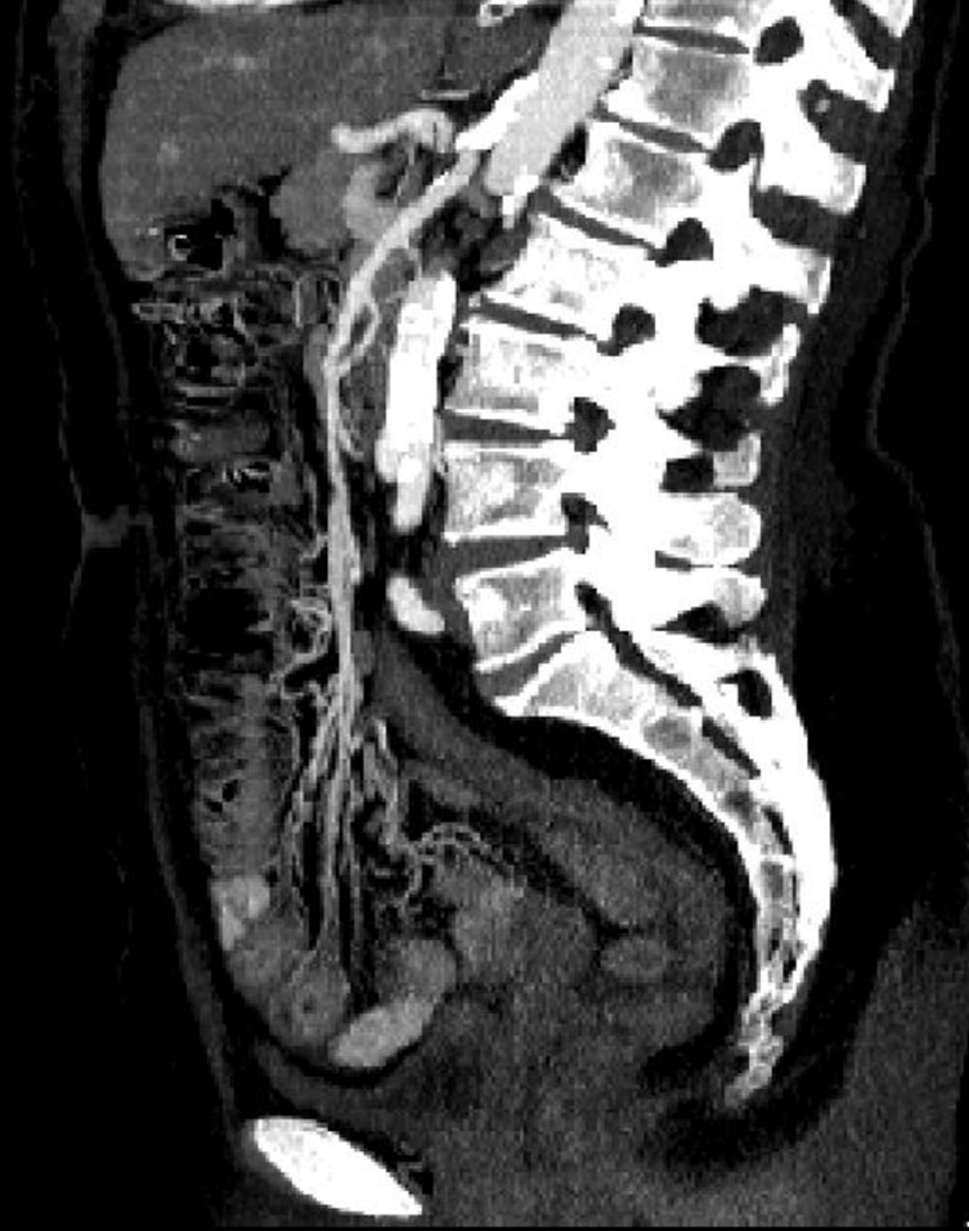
Acute arterial mesenteric ischemia. Contrast-enhanced MDCT 2D reconstruction on sagittal plane in early phase: the CT shows the presence of emboli or thrombi as filling defect in the lumen of the artery. If they are small and peripherally localized, the identification can be difficult. The loops of injured small bowel are contracted in consequence of spastic reflex ileus and intestinal wall shows lacking of/poor enhancement. The mesentery is bloodless, due to reduction in caliber of the vessels and apparently in number.
